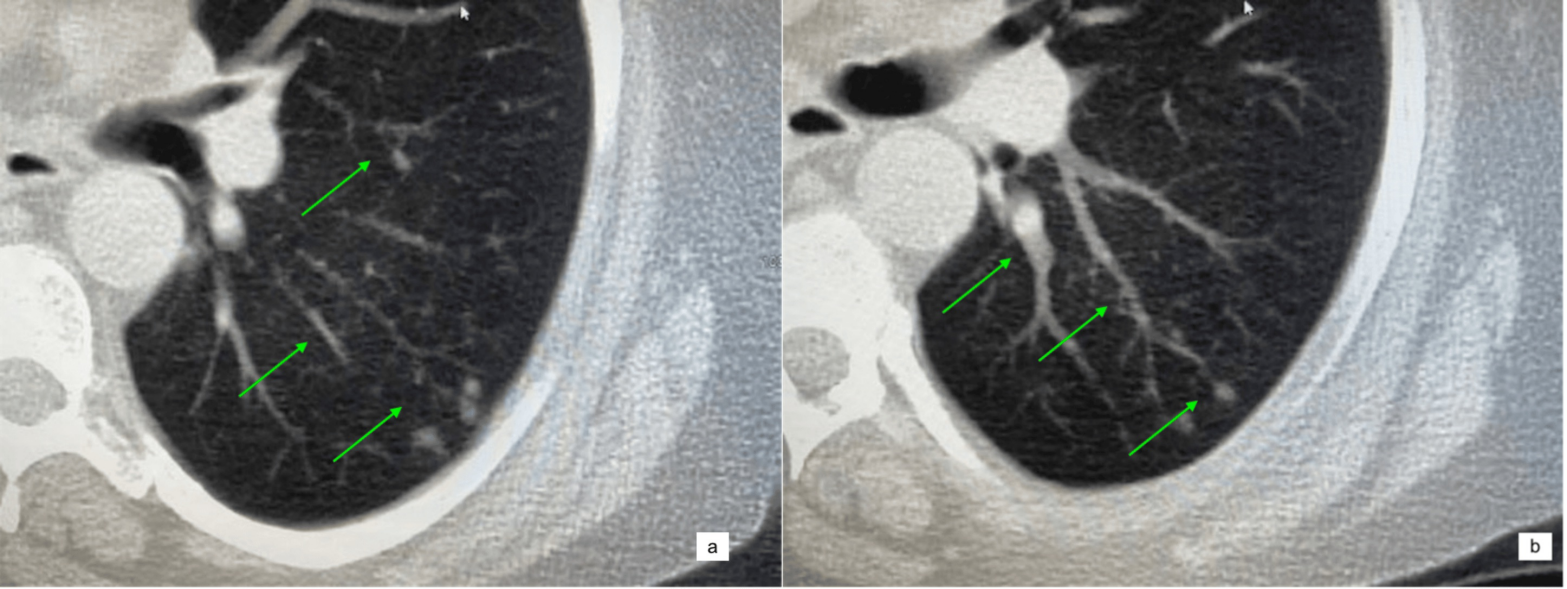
(a, b) CT chest taken on 05/26/21 showing an increased cluster of micronodules in the left base (green arrows) CT: computed tomography
